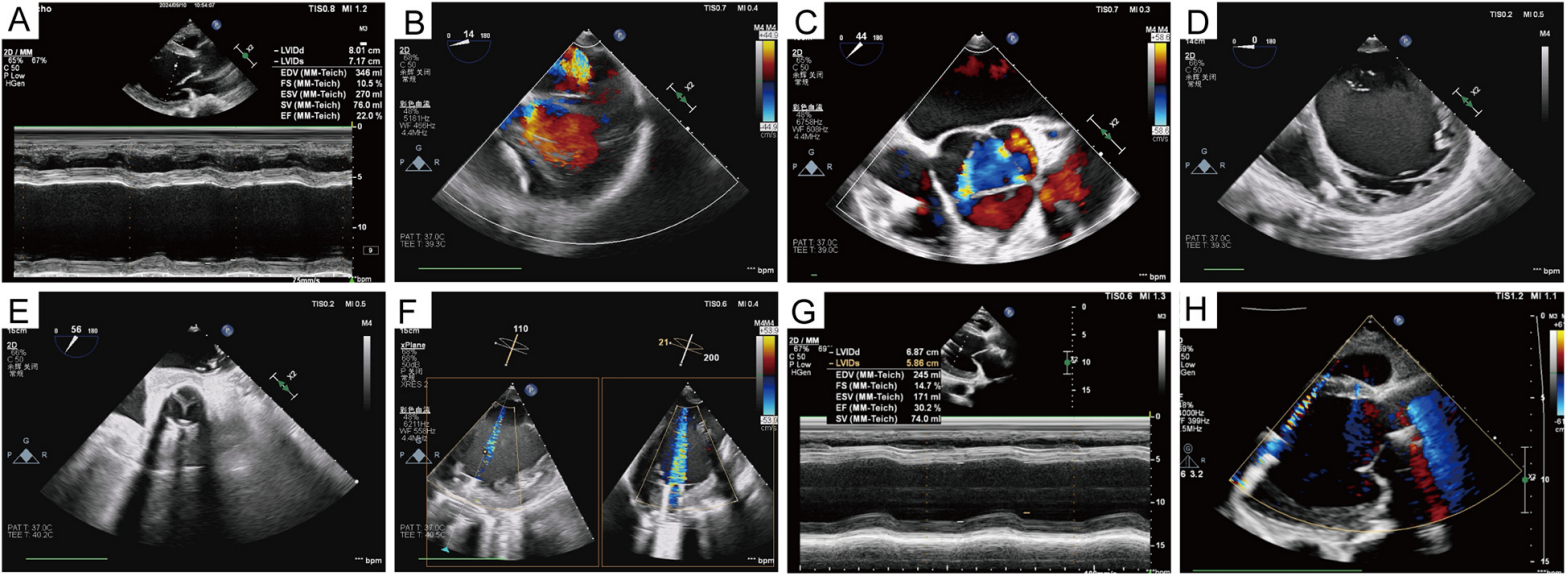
Echocardiographic data. (A) Preoperative ejection fraction and left ventricular diameter. (B–D) Mitral, aortic valve regurgitation, and left ventricular volume before left ventricular assist device implantation. (E) Opening and closing of the prosthetic valve. (F) The relationship between the inflow cannula of the left ventricular assist device and the mitral orifice and the ventricular septum was also demonstrated. (G,H) Left ventricular volume, as well as valve regurgitation, were significantly improved 1 year after the surgery.
